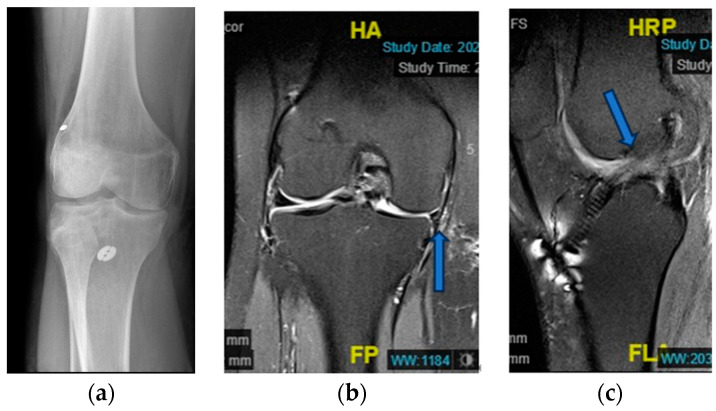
(a) Knee X-ray from local hospital; (b) MRI coronal view of knee joint, with significant medial meniscus loss (arrow); (c) MRI sagittal view of knee joint, ACL graft tear was noted.
